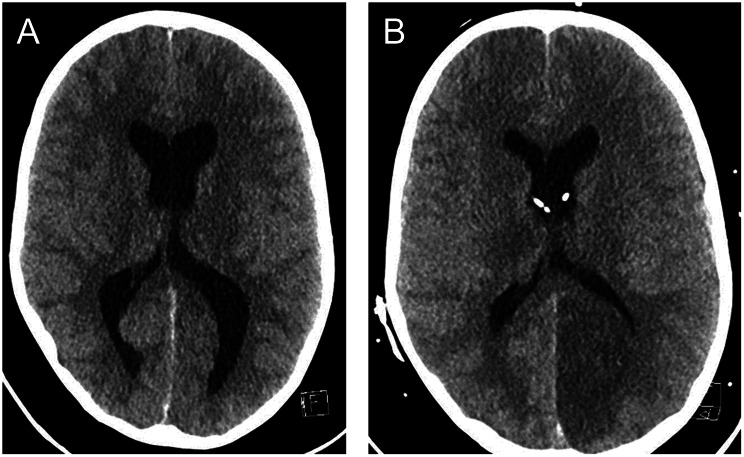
(A) a CT scan of the brain on day 6 showed general oedema, hydrocephalus, and incipient herniation. External ventricular drainages were urgently inserted bilaterally. (B) a postoperative CT scan showed residual mass effect, herniation, and development of multiple infarctions
